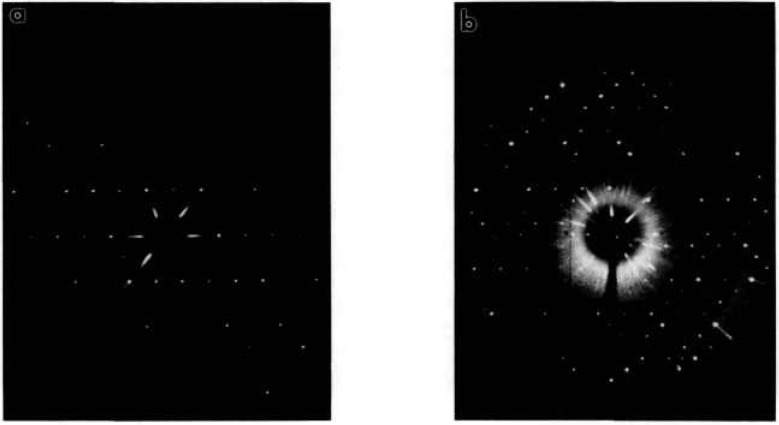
X-ray precession photographs of CaBi_2_O_4_ (Mo radiation) (a) (*h0l*), (b) (*hll*).
